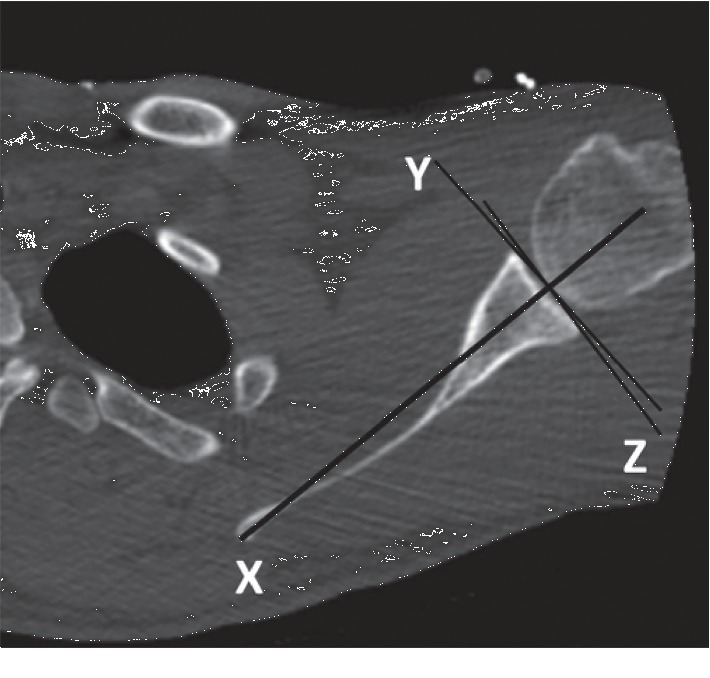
Measurement of the glenoid version on the axial CT image. *X*: scapular axis (Friedman line); *Y*: line of neutral version; *Z*: glenoid axis. The version angle is the angle formed between the *Y* and *Z* lines.
